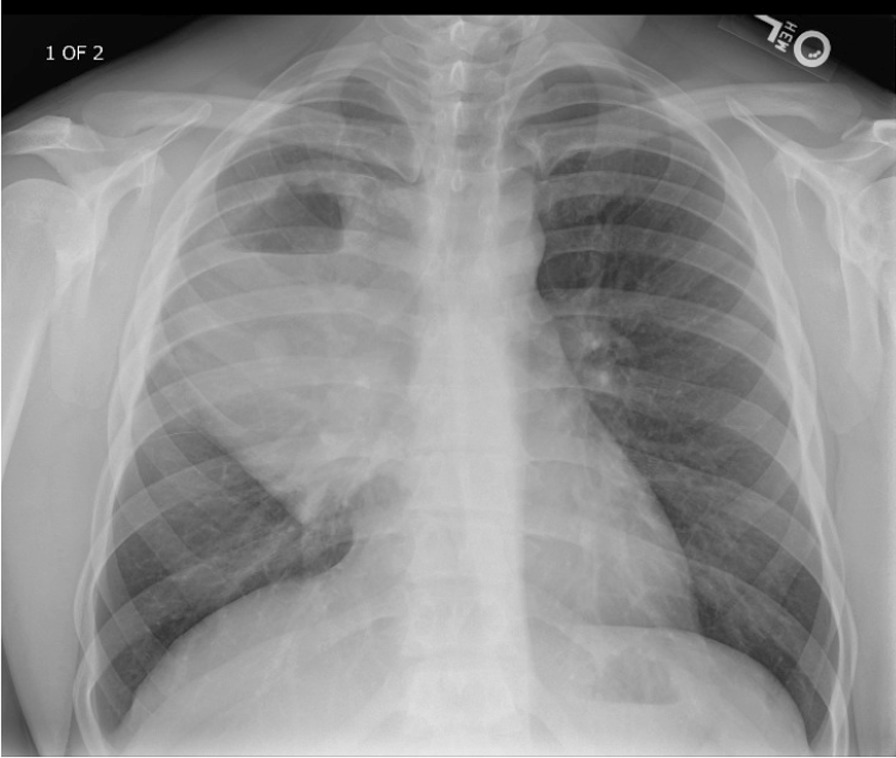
Follow-up chest X-ray (PA view). Enlarging right upper lobe cavitary lesion
